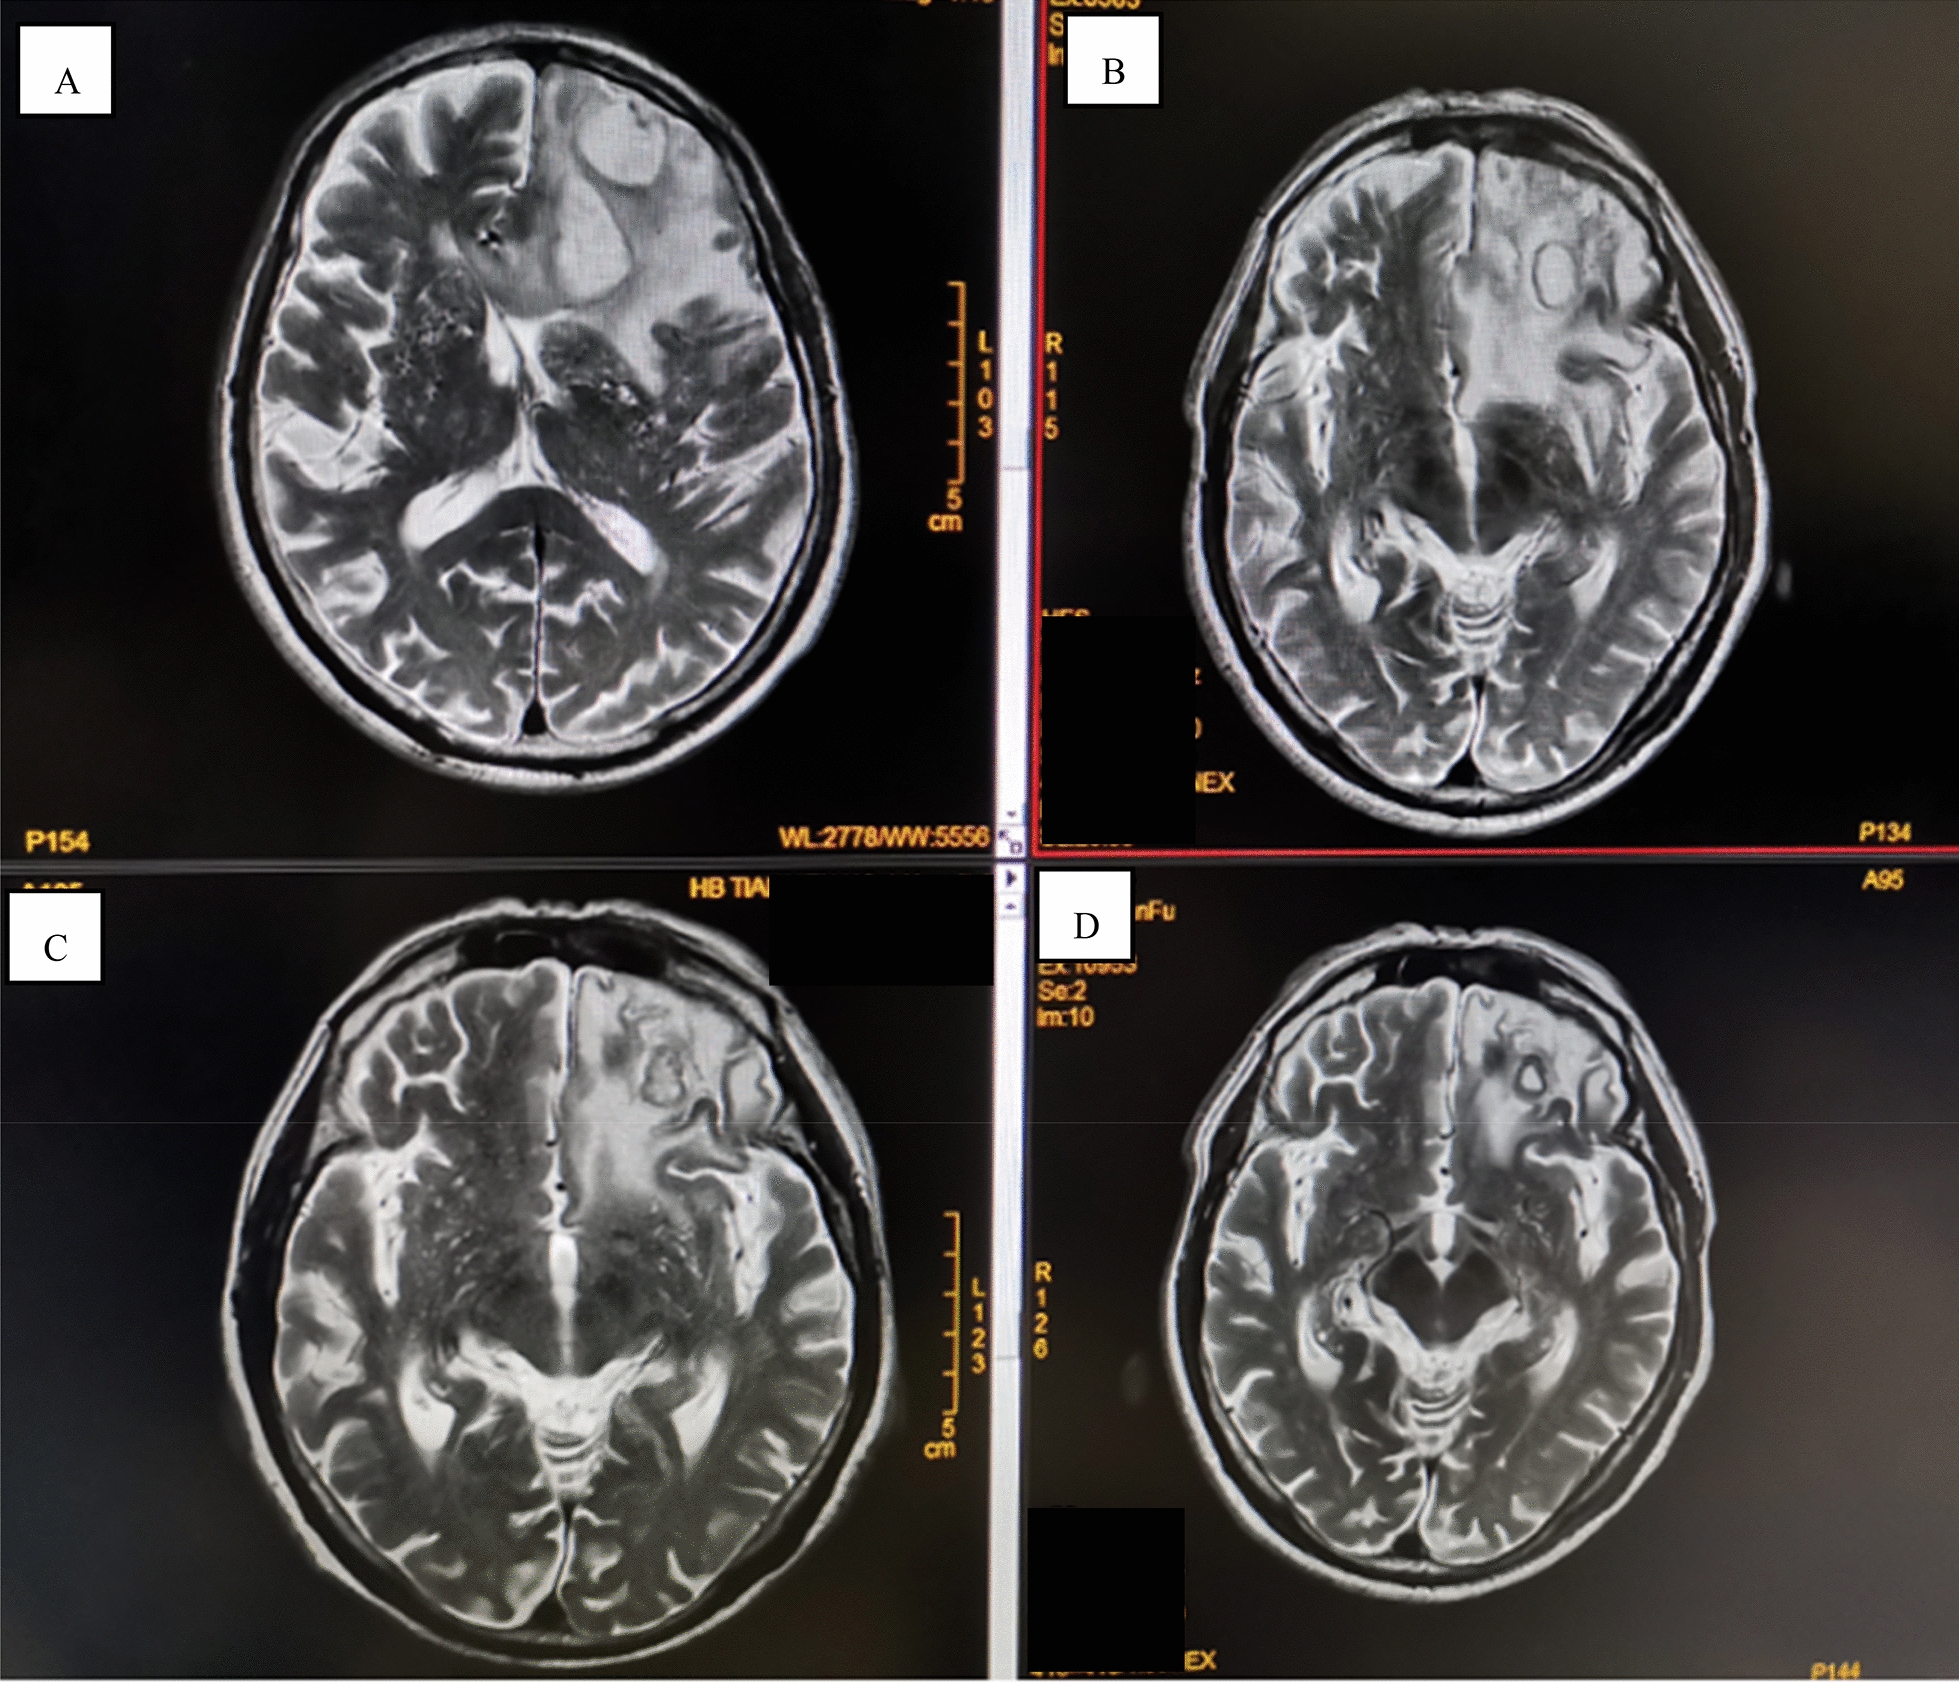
Brain MRI images of the patient. A Brain MRI on August 23. B Brain MRI September 14. C Brain MRI on October 22. D Brain MRI on November 22
